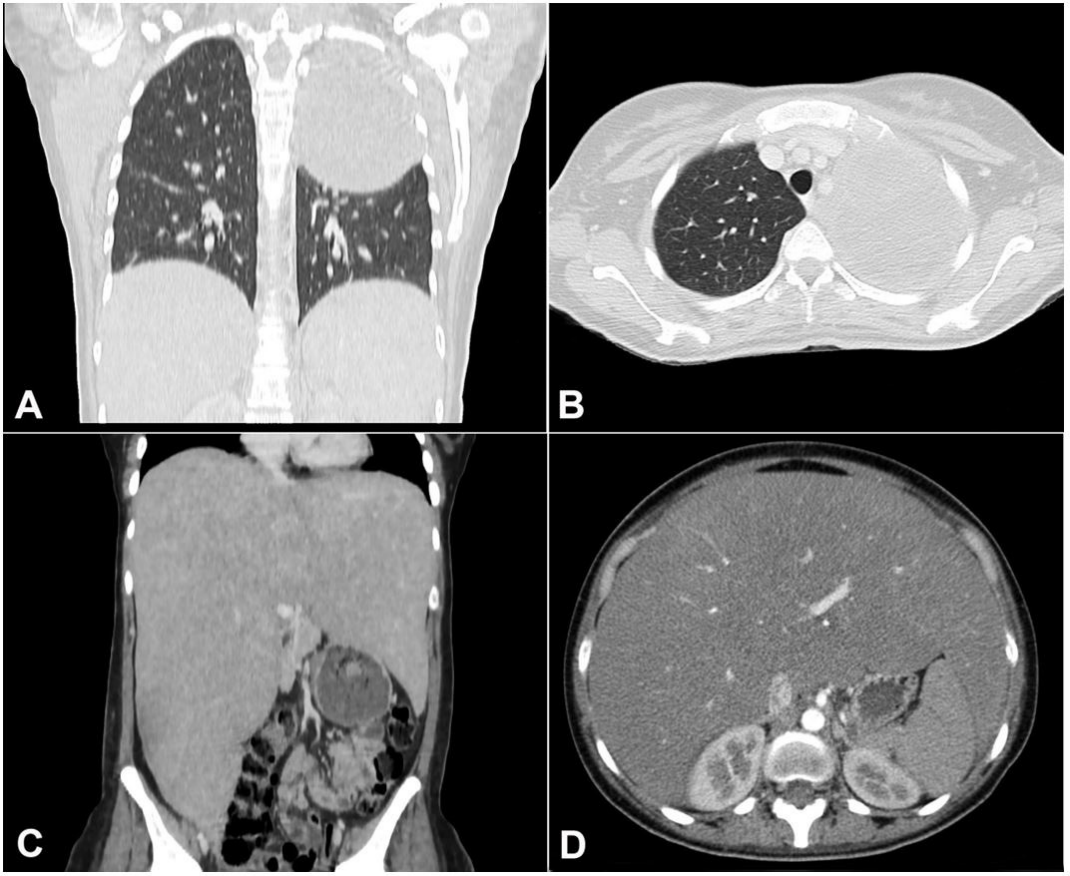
A – Chest CT scan Coronal plane – and B – axial plane -revealing a large mass in the left hemithorax; C – abdominal CT scan coronal plane and D axial plane - showing remarkable hepatomegaly.
